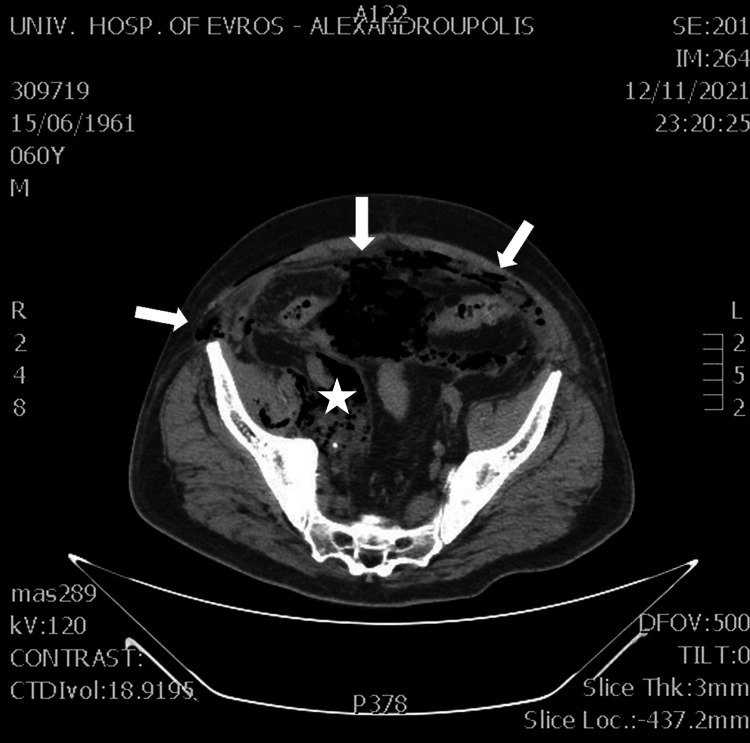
CT examination of the abdomen revealed free air in the peritoneal cavity (white arrows) and the right psoas muscle (white star). CT: computed tomography.
